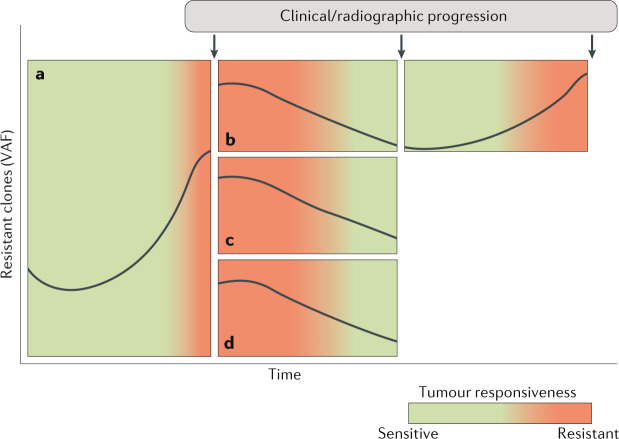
Tumour dynamics of patients receiving biomarker-selected targeted therapies. Patients with *RAS*/*RAF*-wild-type, HER2-negative colorectal cancer receiving anti-EGFR antibodies demonstrate growth of clones of resistant cells over time, as reflected by increases in the variant allele frequency (VAF) of the resistance mutations present in these clones in circulating tumour DNA (ctDNA) (part a). Of note, the emergence of such aberrations in ctDNA typically predates radiographic or clinical disease progression. Such patients can be managed in several ways, including a ‘holiday’ from the targeted agent during the next line of therapy followed by the subsequent re-introduction of the original targeted agent based on a reduction in the VAF of resistance mutations on ctDNA-based monitoring (part b); by introducing a different biomarker-selected targeted therapy or an agent targeting treatment-emergent mechanisms of resistance, as determined by ctDNA findings (part c); or the introduction of one or more agents with activity against other downstream targets (part d).
